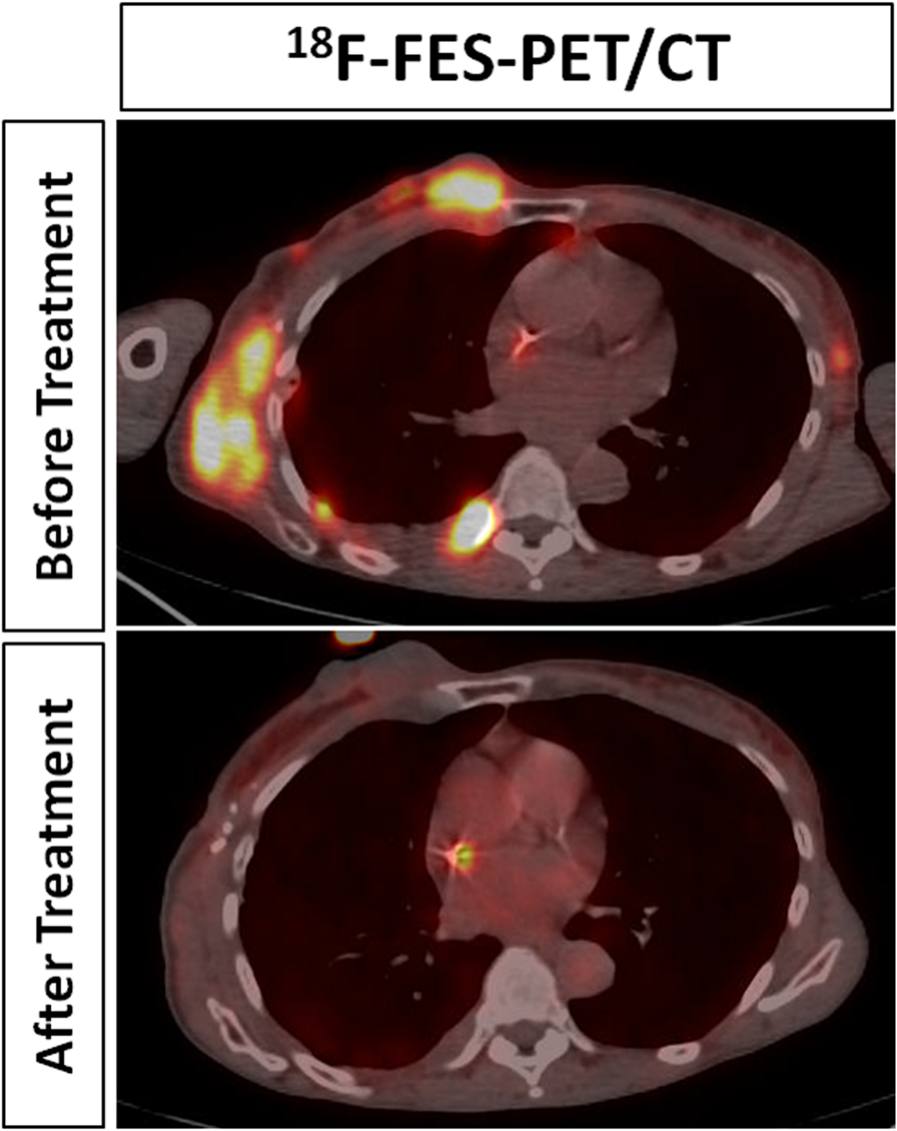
In-vivo monitoring of drug-target-interaction with ^18^F–Fluoro-Estradiol (FES)-PET/CT in a patient with metastatic estrogen receptor-positive breast cancer being treated with an estrogen receptor-directed agent. The pre-therapy scan (top image) showed multiple chest wall metastases with avid tracer uptake. After initiation of the therapy (bottom image), tracer uptake resolved, while the size of the metastases did not change. It is important to recognize that these findings prove that the drug has hit its target but do not necessarily indicate treatment response. Retained tracer is seen in a central venous catheter on both studies
